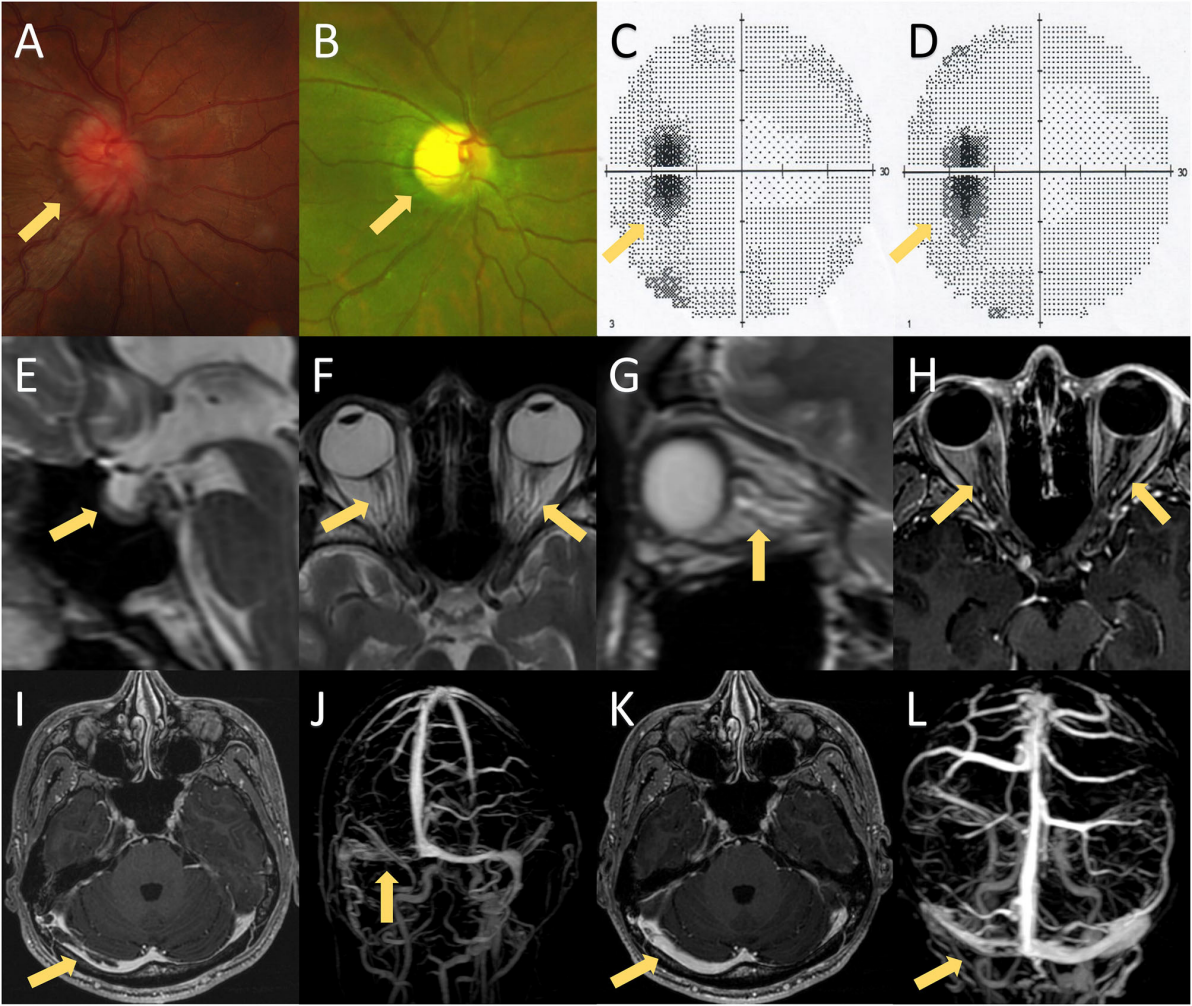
MRI findings, fundoscopic findings, and visual fields of the presented case. (A) Fundoscopic examination on the first hospitalization showed bilateral papilledema. (B) Fundoscopic examination on the second hospitalization revealed bilateral optic atrophy, which was probably caused by bilateral papilledema. (C,D) Visual fields on the first and second hospitalizations showed bilateral enlargement of the physiological blind spot without any other visual field defect. (E–G) Brain MRI exhibited several imaging signs of intracranial hypertension, including the empty-sella sign (E), perioptic subarachnoid space distension (F,G), optic nerve tortuosity (G), and posterior globe flattening (F,G). (H) Brain MRI with contrast showed no enhancement of the optic nerve. (I,J) Post-contrast 3D GRE T1-weighted imaging and contrast-enhanced magnetic resonance venography (CE-MRV) showed filling defects of the right transverse-sigmoid sinus. (K,L) Brain MRI and CE-MRV after 3 months of anticoagulation showed partial recanalization of the right transverse-sigmoid sinus.
